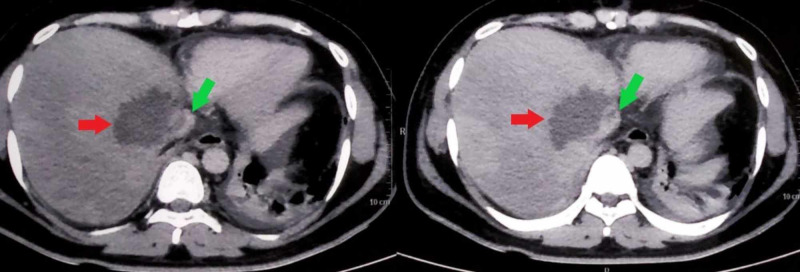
Axial sections of computed tomographic (CT) scan of abdomen showing the liver abscess (red arrows) and thrombus in the adjacent inferior vena cava (green arrows).
